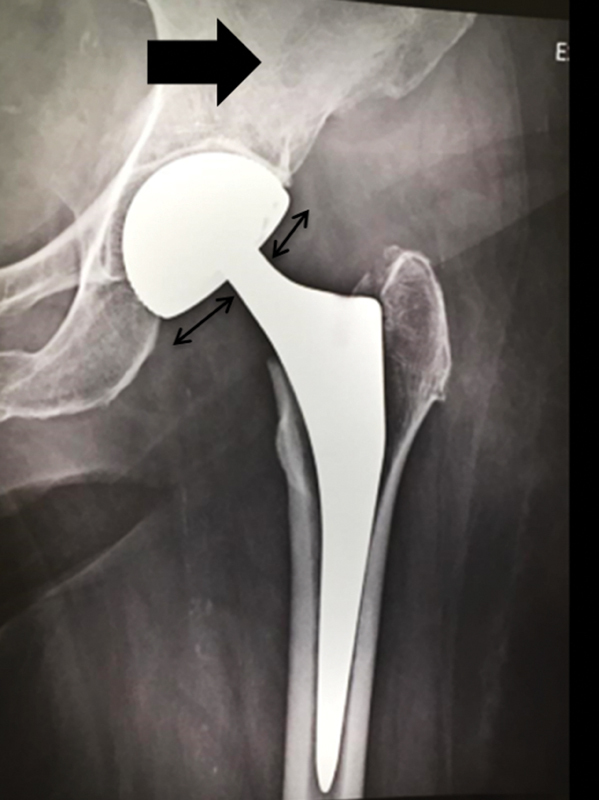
X-ray 7 days after reduction. The larger arrow shows the "bubble sign", the polyethylene displaced in the soft parts. The smaller arrows show the eccentricity of the metal head in the acetabular dome.
